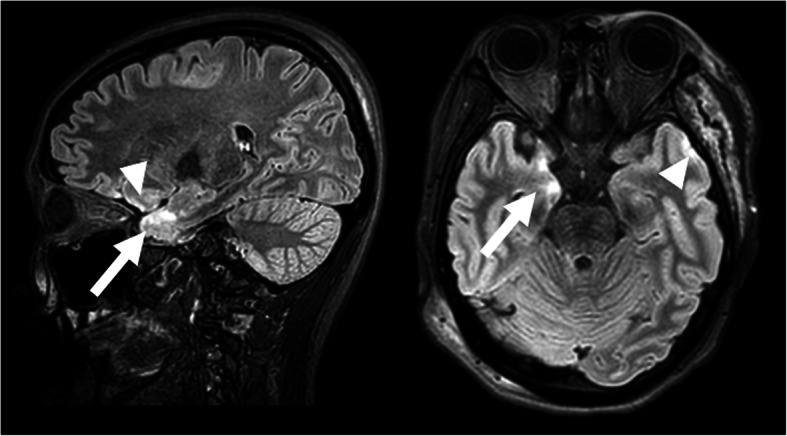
Fluid attenuated inversion recovery (FLAIR) imaging of head magnetic resonance imaging (MRI) in the same patient with cerebral dyschromatopsia. Right figure is a sagittal image, and left figure is an axial image at 3 days after injury. There were signs of brain contusion in the right anterior temporal lobe (arrows) and the presence of subarachnoid hemorrhages (arrowheads)
